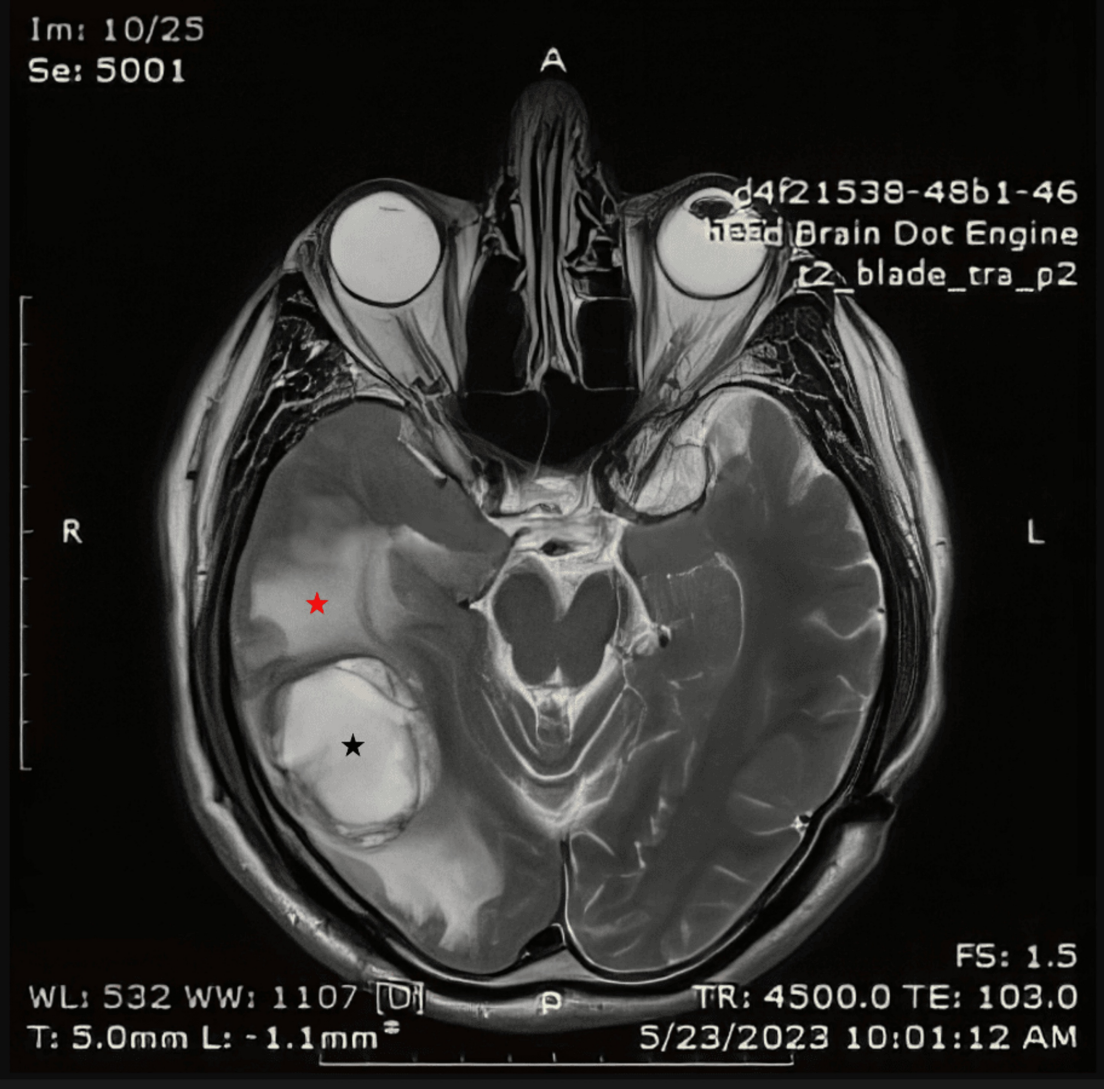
T2-weighted image The MRI image shows a hyperintense mass originating in the right temporal lobe (black star) with edema of the surrounding tissue (red star)
